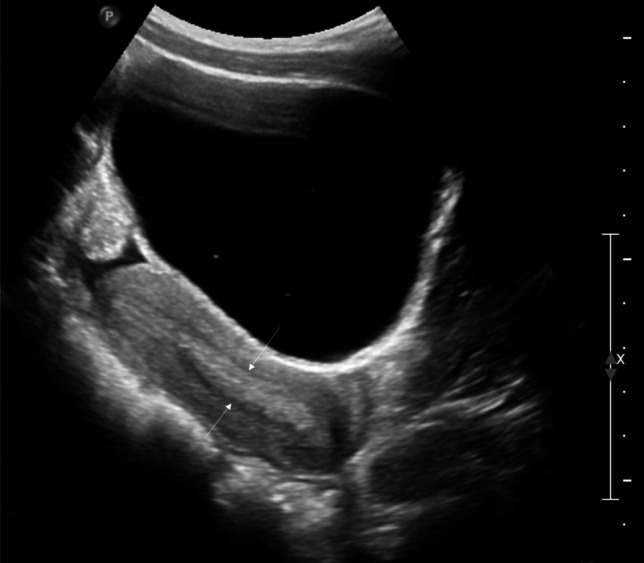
Sagittal gray-scale pelvic ultrasound in the same patient as in Fig. 1 shows a large stimulated uterus with a thick endometrium (*arrows*)
